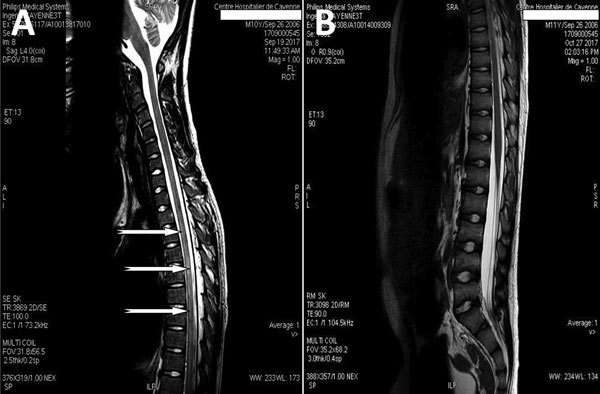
Magnetic resonance imaging (MRI) of the spine in a 10-year-old boy from Brazil with *Angiostrongylus cantonensis* infection. A) MRI before treatment showing myelitis; sagittal T1 postcontrast sequences show intramedullary enhancement in the thoracic spinal cord T2–T10 with diffuse leptomeningeal enhancement (arrows). B) Normal MRI 1 month after treatment.
